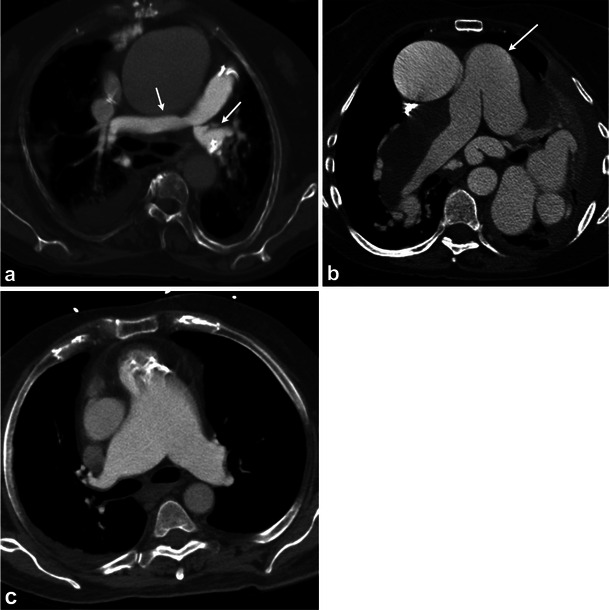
Contrast-enhanced axial CT image (a) from a patient with tetralogy of Fallot (*TOF*) and a prosthetic pulmonic valve demonstrates severe stenosis of the left and mild stenosis of the right pulmonary artery (*arrows*). In addition there is an ascending aortic aneurysm. In a different patient (b) with an unrepaired *TOF*, an aneurysm of the pulmonary trunk (*arrow*) formed with a chronic thrombus in the right and left pulmonary arteries. Also note the multiple dilated aortopulmonary collaterals. CT angiogram c performed with 30 ml of contrast in a patient with chronic renal failure and a prosthetic pulmonary valve demonstrates a main pulmonary artery aneurysm (measuring 44 mm)
